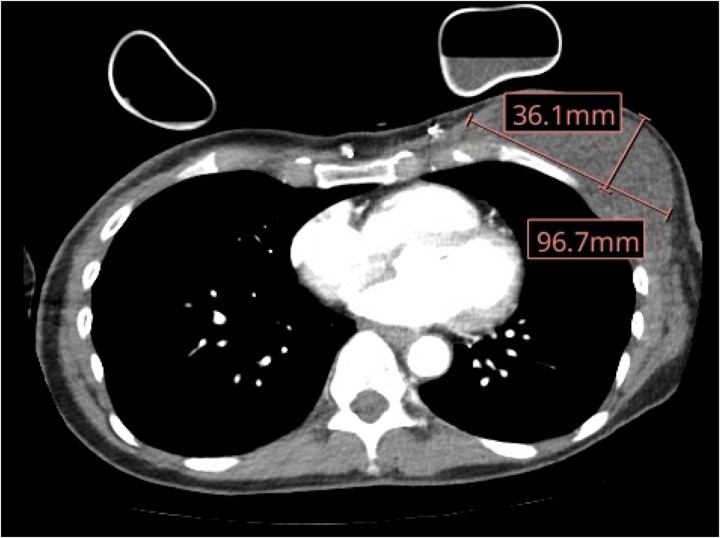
CT chest with IV contrast demonstrating a 9.6 × 3.6 cm fluid collection in the left mastectomy bed.
